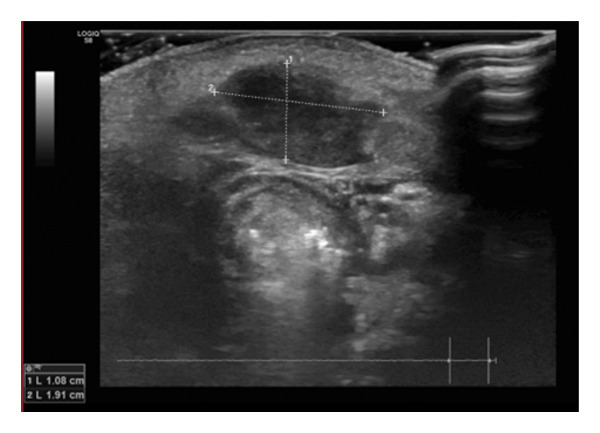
Ultrasound examination of the submandibular gland showing a longitudinally oval mass at the site of the submandibular gland, size 1.1–1.9 cm, anechoic in the center, with peripheral vascularity and an echogenic surrounding reaction.
